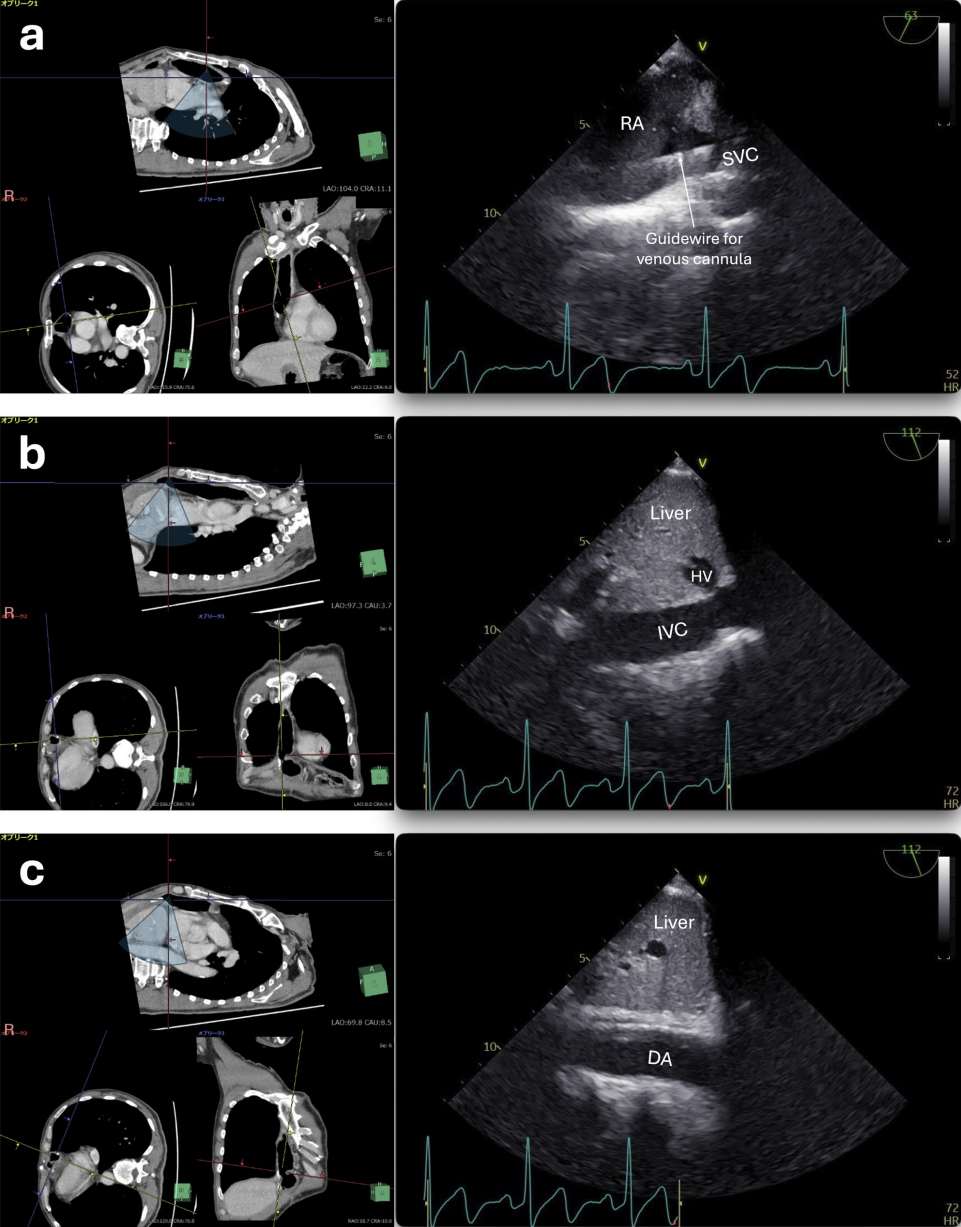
Transesophageal echocardiographic images, similar to right parasternal and subcostal views, and corresponding sections on computed tomography. The right pane of each subfigure shows transesophageal echocardiographic images, while left pane shows corresponding sections on preoperative computed tomography (CT). a Bicaval view with a guidewire in the superior vena cava, b Subcostal view of the inferior vena cava, c Subcostal view of the descending aorta. The inclinations of the yellow lines in the bottom-left and bottom-right CT images indicate the probe rotation and transducer array angles, respectively, when obtaining ultrasound images corresponding to the top CT images. The blue-shaded fan shapes in the top CT images replicate the ultrasound scanning area. DA, descending aorta; HV, hepatic vein; IVC, inferior vena cava; RA, right atrium; SVC, superior vena cava
